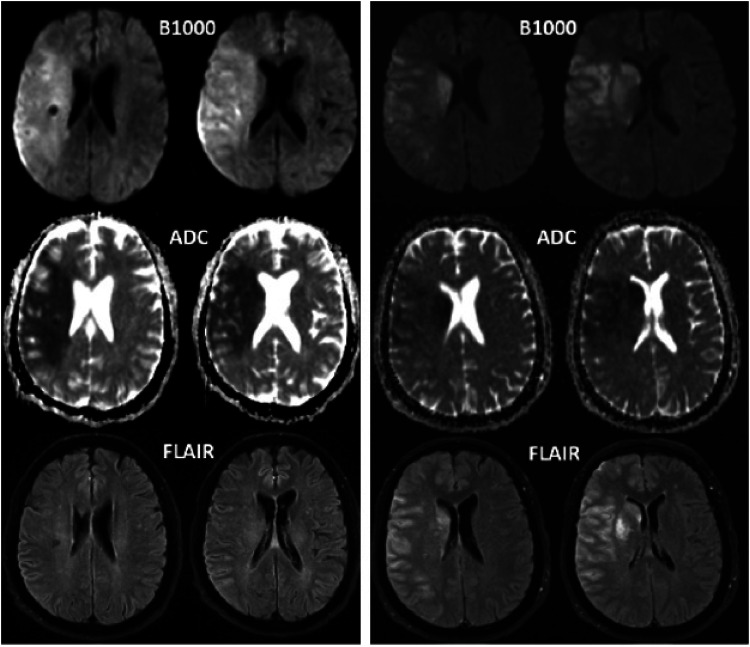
Baseline (left) and post-thrombectomy (right) MR images in a 69-year-old patient who initially presented with acute onset left-sided weakness and slurred speech. She was found to have right ICA occlusion with ischemic core volume calculated at 184 mL. The patient underwent successful reperfusion (mTICI 2b), and post-thrombectomy imaging revealed a significantly smaller infarct volume of 140 mL on B1000 images. ICA: internal carotid artery.
